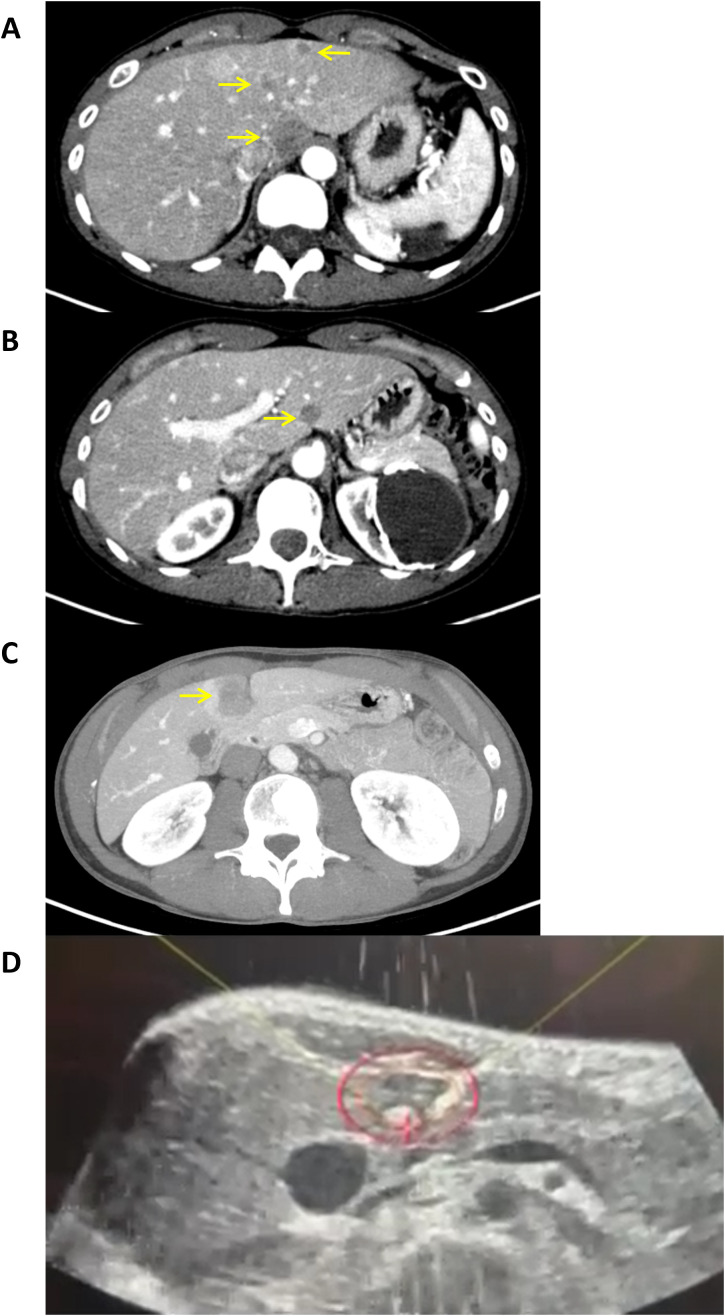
(A–C) Axial contrast-enhanced CT scans of liver before histotripsy treatment displaying multifocal breast cancer metastases in the left lobe. Target tumor lesions in segments 2, 3, and 4 are identified by arrows. (D) Intraprocedural ultrasound image of defined treatment volume within the red circle encompassing the hyperechoic targeted tumor. The focal point of the transducer is the red crosshair where the treatment effect is visible as an echogenic bubble cloud.
